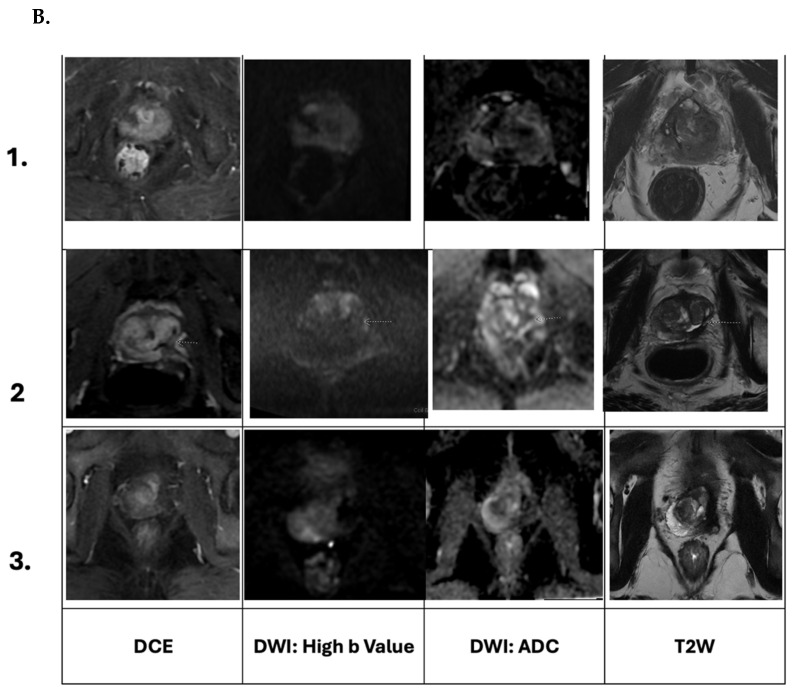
(A) Stepwise algorithm for characterizing lesions within the ablation zone following focal therapy based on a 3-point PI-FAB scoring system. In the first step, DCE is the most important sequence. In the second step, findings should be compared to the pre-focal therapy MRI to assess for a potential upgrade or downgrade of the PI-FAB score. (B) Examples of MRI scans after focal therapy, with varying findings within the ablation zone. Row 1: PI-FAB 3: MRI performed 12 months after right hemiablation with HIFU. At the anterior aspect near the apex, there is a focal lesion with early enhancement (DCE +), corresponding high-b images (DWI +), and restricted diffusion on ADC (+), as well as hypointensity on T2WI (+) corresponding to the prior lesion seen in prior exam. This would give an overall PI-FAB score of 3. Targeted biopsy of this lesion was positive for 7 of 12 cores, revealing grade group 3 cancer with a maximum cancer core length of 12 mm. Row 2: PI-FAB 2: MRI performed 9 months after right peripheral zone HIFU. At the posterolateral peripheral zone there is a focal lesion outlined by the dotted arrow in dynamic post contrast, DWI, ADC and T2W respectively showing mild enhancement in DCE (+), no signal in DWI (-), and positive hypointensity in ADC (+) and T2WI (+). This would give an overall PI-FAB score of 2. Targeted biopsy of this lesion was negative for malignancy. Row 3: PI-FAB 1: MRI performed 7 months after left-quadrant ablation with HIFU. There is no contrast enhancement within the ablation zone on DCE (-), T2 focal hypointensity, and diffusion restriction DWI (-), giving an overall PI-FAB score of 1. Targeted biopsies sampling the whole prostate were all negative. ADC= apparent diffusion coefficient; DCE = dynamic contrast-enhanced; DWI = diffusion-weighted imaging; HIFU = high-intensity focused ultrasound; T2W = T2-weighted.
